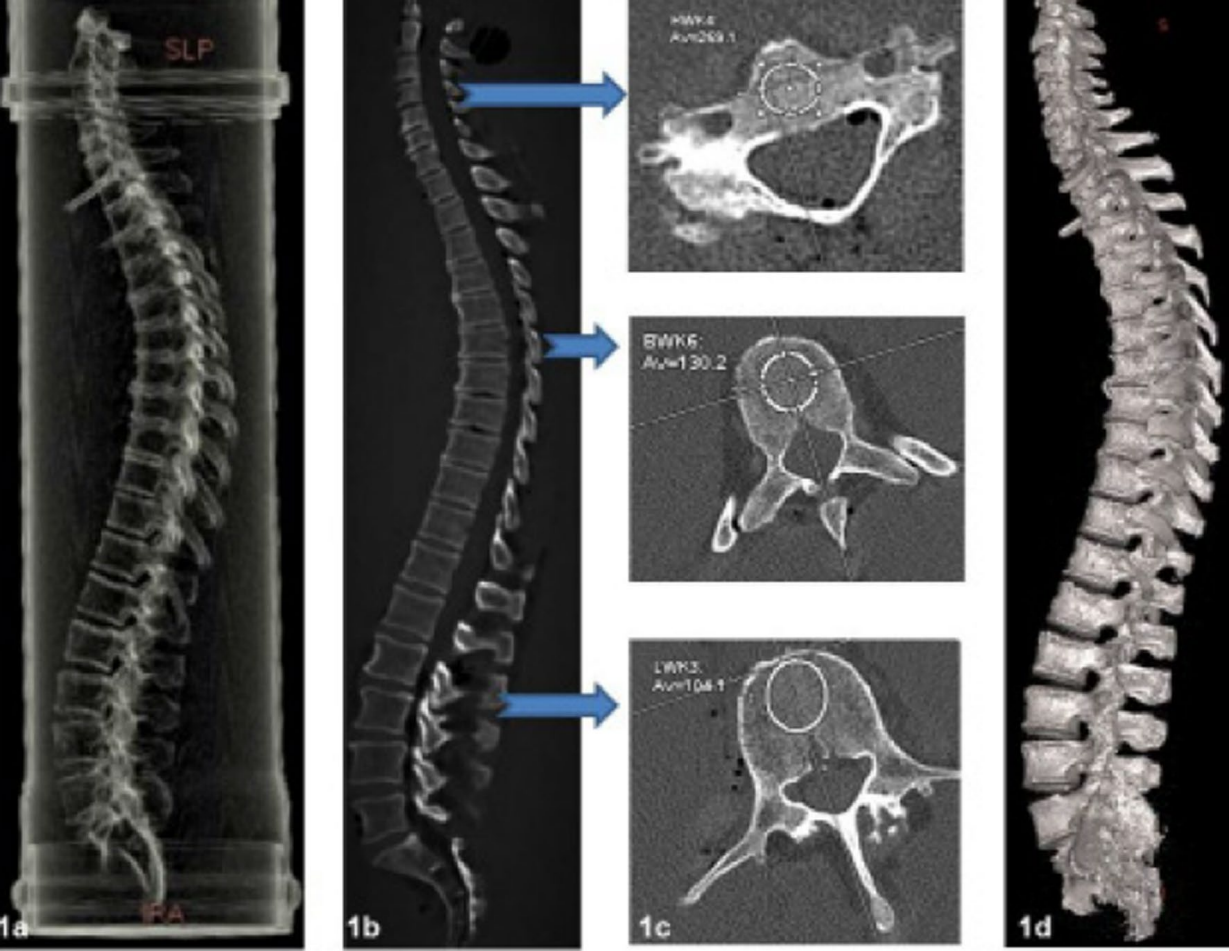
Experimental setup, image reformation, and measurements. 1a The location of an embedded spine in the PVC tube using a transparent 3D reconstruction. Sagittal reconstructions were obtained from the axial CT scans to detect fractures (1b). An ROI was positioned mid-vertebrally in each vertebral body, and the density was determined in HU (1c). A 3D reconstruction allowed final assessment of deformities and fractures (1d)
